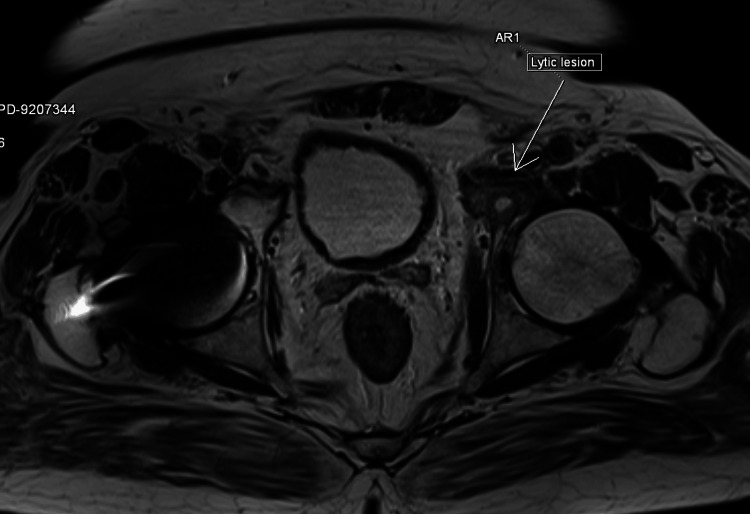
CT-MRI of the pelvis with bilateral hips revealed an osteolytic soft tissue lesion involving the left acetabulum
